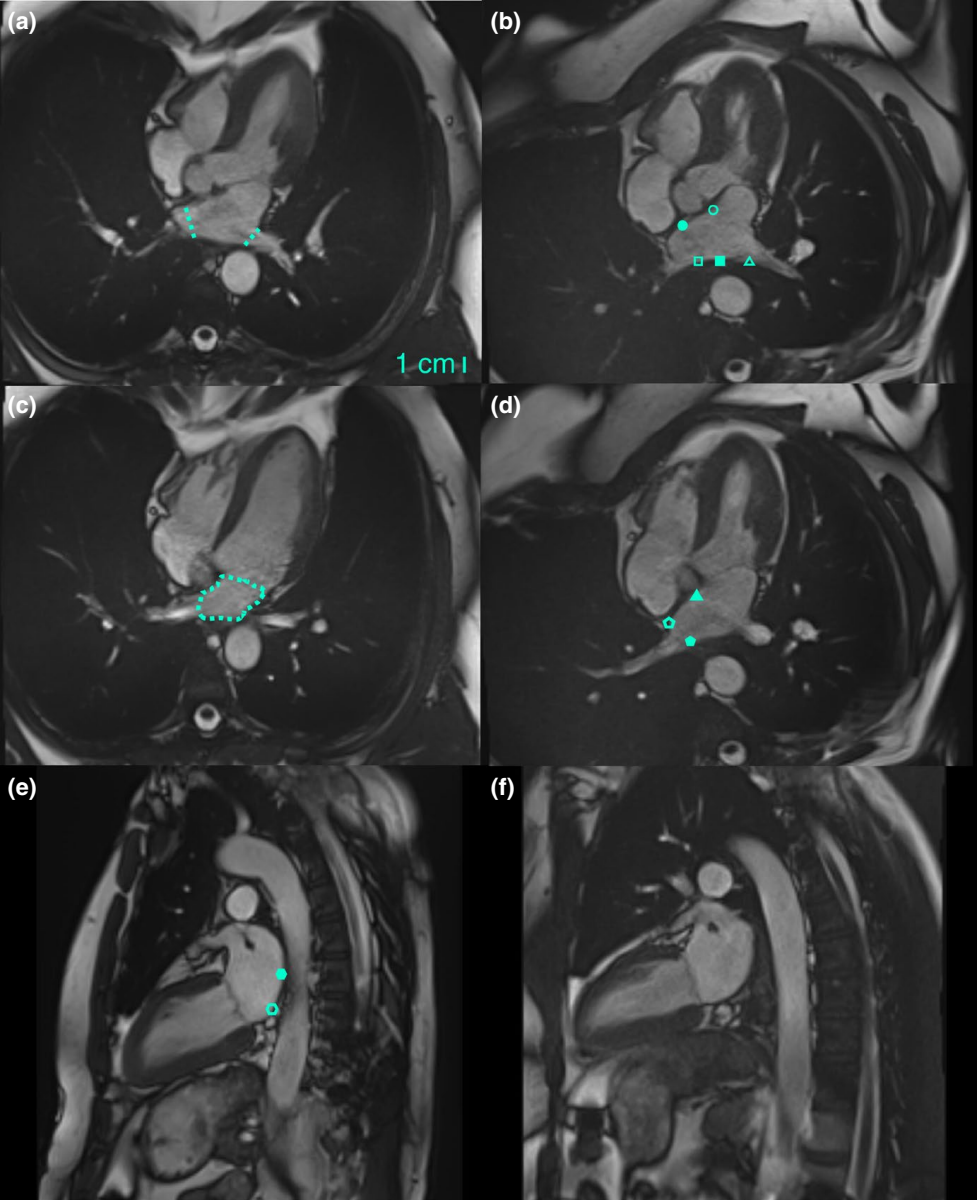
Representative 2‐ and 4‐chamber MRI in supine and LL position. (a) Four‐chamber MRI with RSPV and LSPV in supine position. Dotted lines indicate the PV diameters at the ostium. (b) Image in LL position with RSPV and LSPV. Regions for strain analysis were a LA septal (open circle), a RSPV anterior (closed circle), a RSPV posterior (open square), a LA posterior (closed square), and a LSPV posterior wall (open triangle). Note the sharp curvature of the LSPV anterior wall toward the LA appendage making tracking imprecise. (c) A 4‐chamber image with RIPV in supine position. Dotted line indicates the LA area used for volume estimation. (d) Image with RIPV in LL position. Regions for strain analysis were a LA septal (closed triangle), an RIPV anterior (open pentagon), and a RIPV posterior wall (closed pentagon). (e) Two‐chamber MRI in supine position. Strain regions were an inferior LA (open hexagon) and a posterior LA wall (closed hexagon). (f) Two‐chamber image in LL position. All images show the moment of maximum LA dilatation immediately before mitral valve opening. Lacking reproducibility of the MRI in the two body positions prevented us from analyzing all PVs
